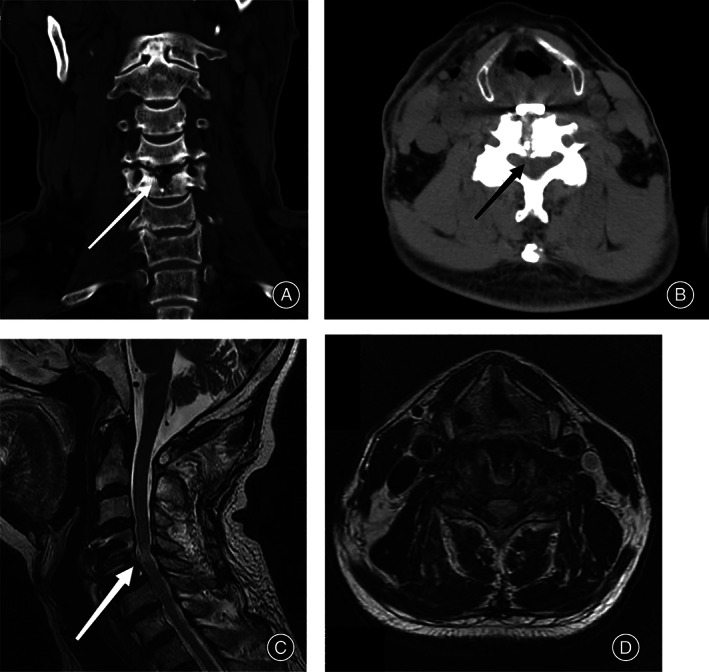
The numbness in the extremities was relieved. Muscle strength in the extremities recovered to Grade 4, and muscle tension improved. The cervical VAS score at 3 days after surgery was 3. The JOA score was 14. The CL angle was 34.99°. The above aspects were better than the preoperative ones. The neck VAS score at 45 days after surgery was 1.9, and the JOA score was 29. The following are the postoperative radiology data: (A) Coronal CT scan 3 days after the surgery showed that there was a bone groove 9.4 mm wide and 7.8 mm long on the C4/5 centrum. (B) In axial CT, the screw fixation was good, the ossification of OPLL was removed, and the OR was 0. (C) At 45 days after the operation, the compression of the spinal cord was relieved according to a sagittal MRI. There was a hyperintense area in the C4/5 level spinal cord. (D) The volume of the spinal canal was recovered, the anteroposterior diameter was 8.1 mm, and the OR was 0. All parameters were significantly better than the preoperative ones
